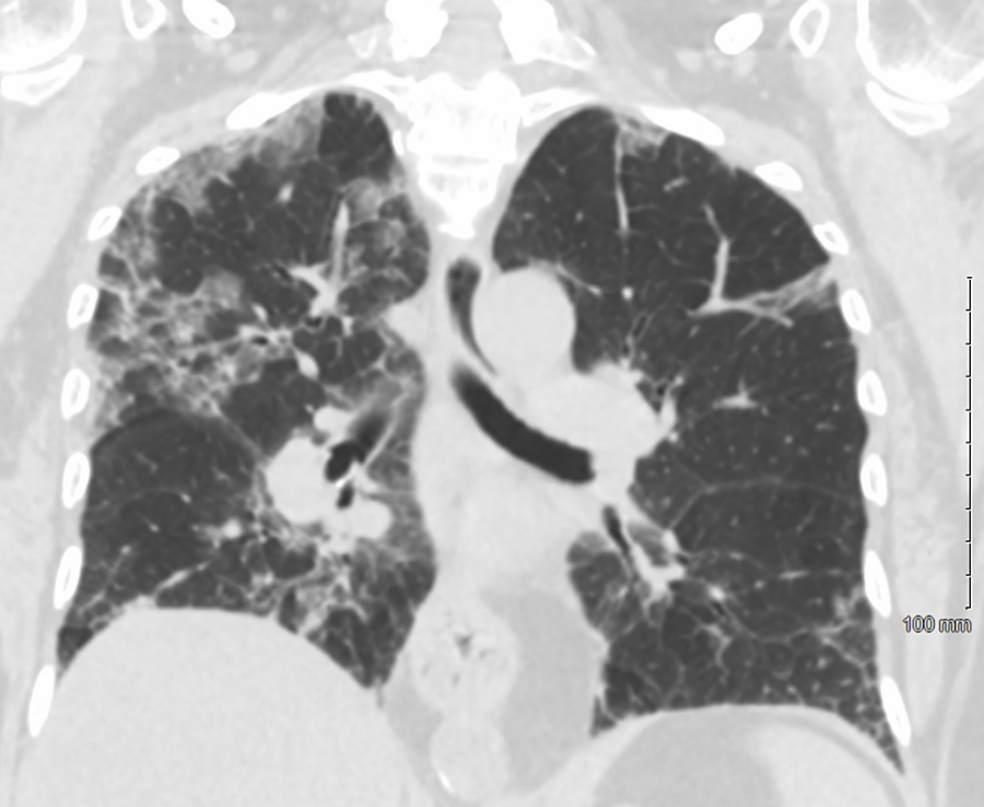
CT coronal lung window, redemonstrating diffuse bilateral ground glass opacities with peripheral predominance and some areas of septal thickening
